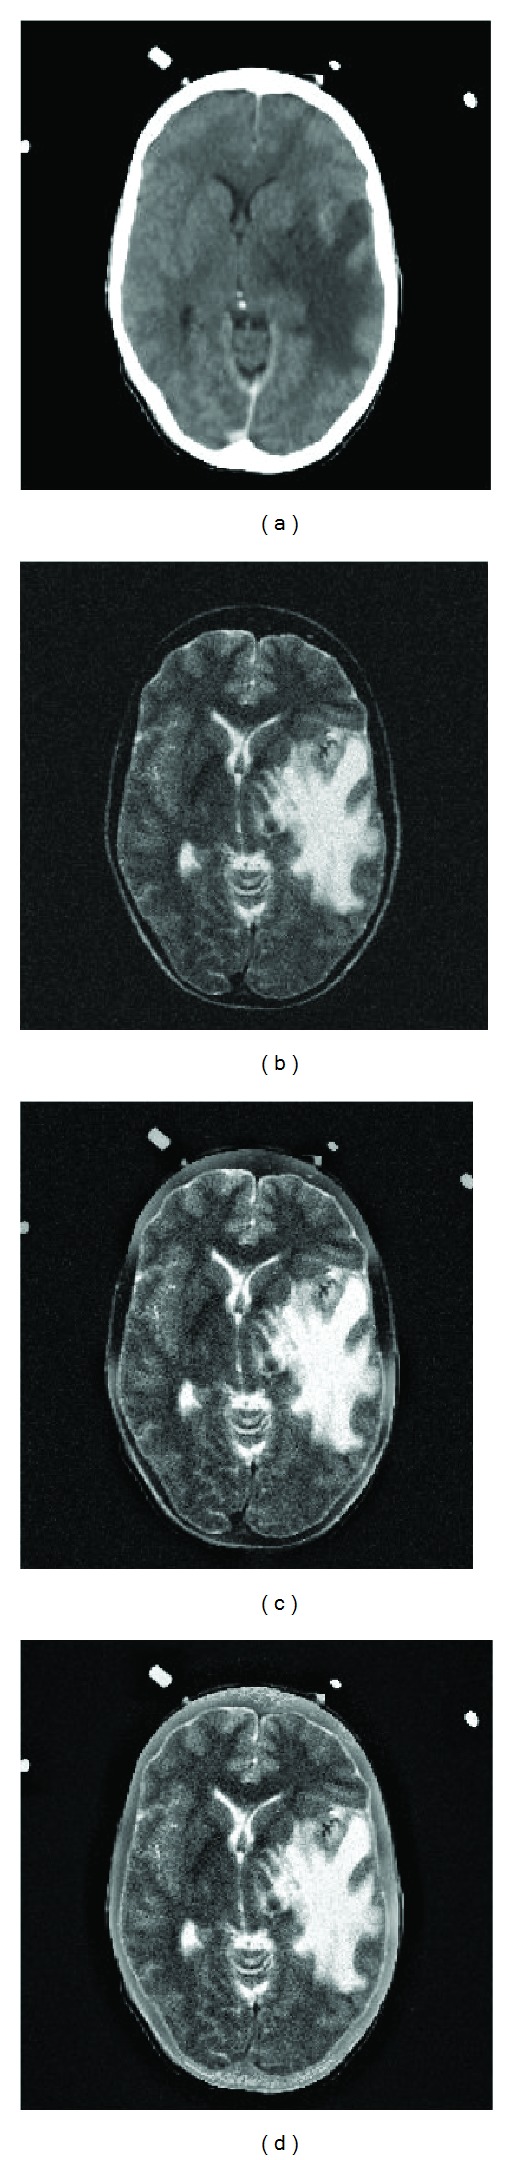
(a) CT image, (b) noisy MR image, (c) GFF [30] fused image, and (d) proposed fused image.
